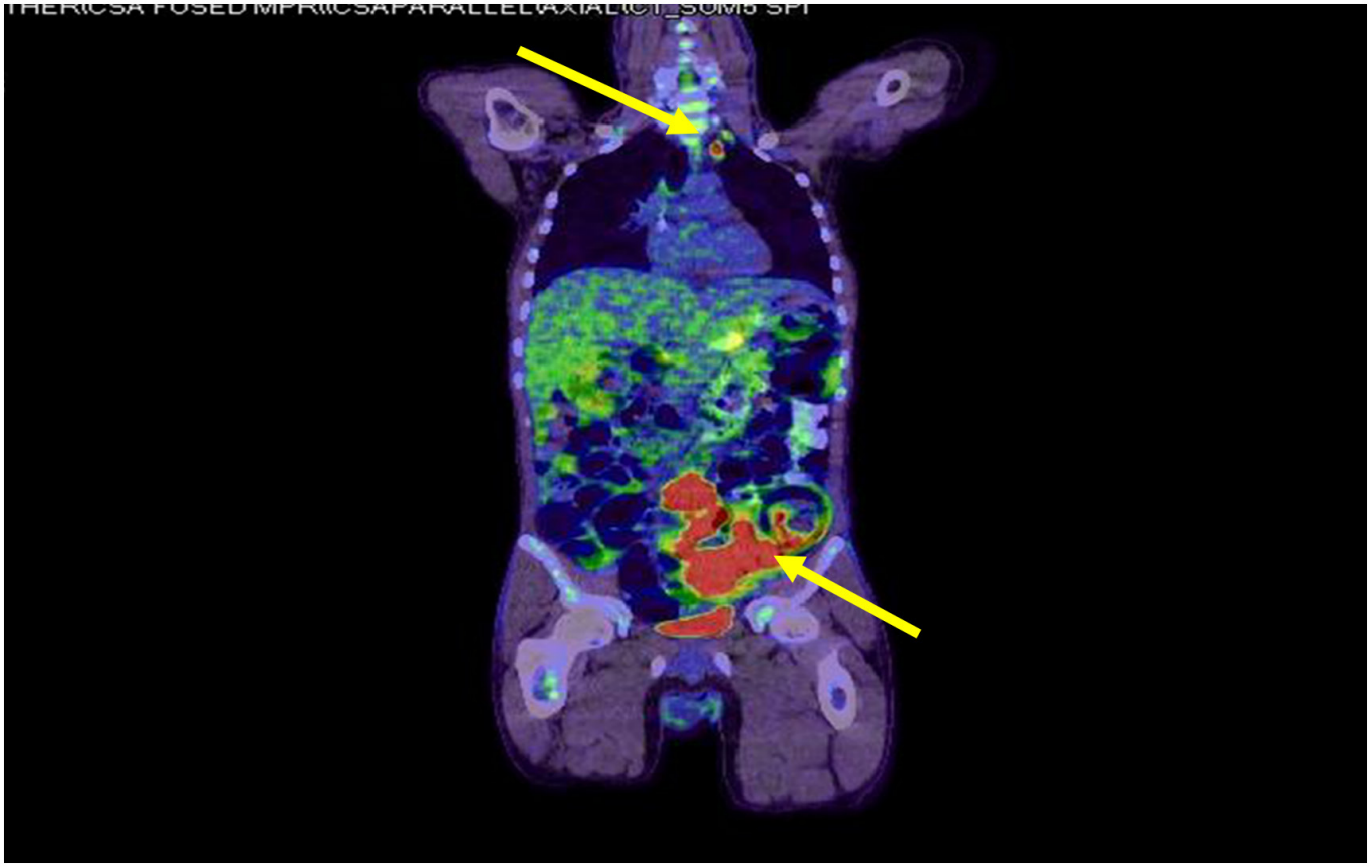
PET/CT showed a thickening of the distal sigmoid colon and distant lymph nodes in the left supraclavicular region.
